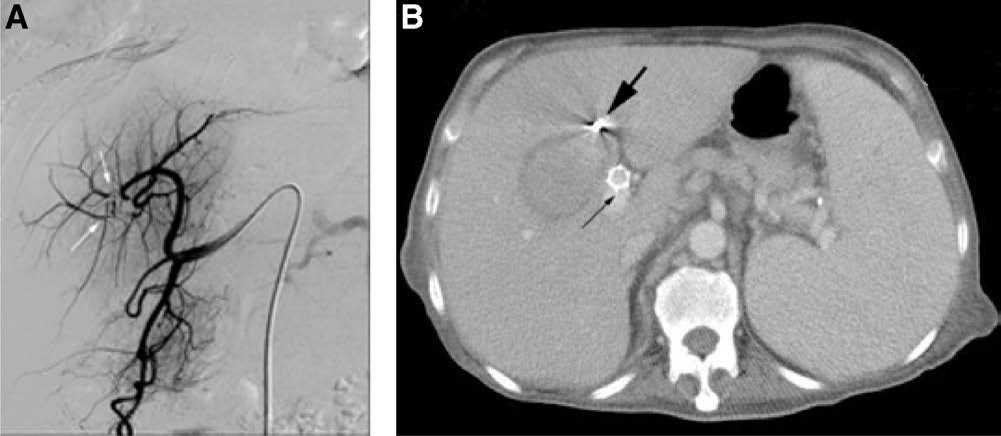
Images after embolization of the pseudoaneurysm with micro coils. (A) Hepatic angiography confirmed embolization of the pseudoaneurysm with micro coils (white arrows). (B) CT scan indicated occlusion of the pseudoaneurysm (black arrow).
